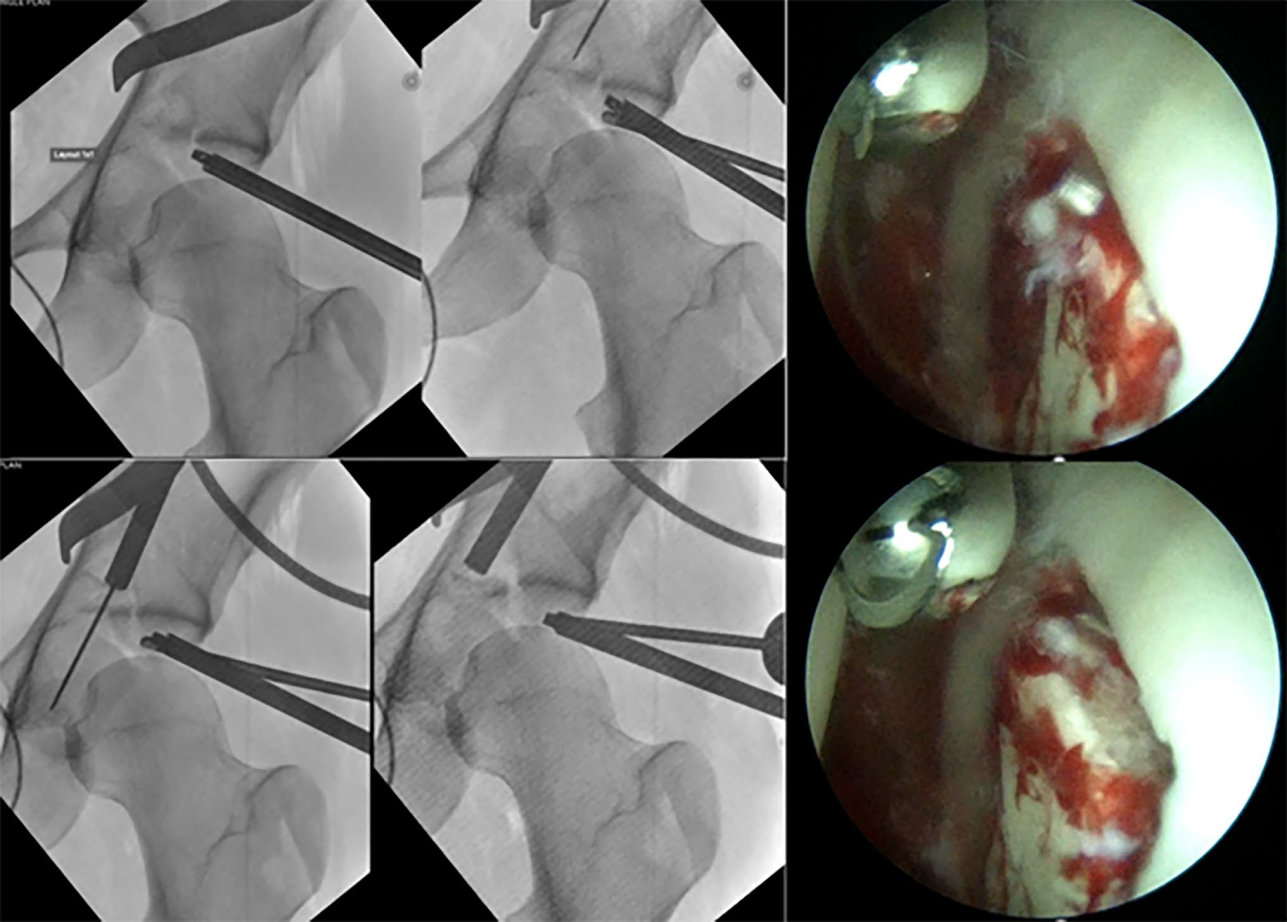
A healthy 21-year-old man presented to our ER after a crush trauma at work under a 500 kilos weight. The X-Ray and CT scan show an anterior column fracture plus posterior hemitransverse compound fracture (ACPHT) with joint depression of the acetabular roof. An AIP approach and hip arthroscopic assistance were performed. The patient was set up in supine position under general anesthesia on a carbon orthopedic traction bed. The fracture of the anterior column was identified through the AIP approach. The medial roof fracture and an important depression of the articular surface were identified through arthroscopic assistance. Supracetabular K-wire was placed under fluoroscopic control and proximal cortical flap was prepared to aid the fragment reduction. A cannulated ram was placed over the K wire and the depressed fragment was pushed stepwise until an anatomic articular reduction is achieved. At the end hypercorrection of the fragment was obtained as planned and synthetic bone was placed to fill the depression. The movement is continuously supervised by the arthroscope. Furthermore, the reduction of the fracture is evaluated by fluoroscopy. Surgery was performed in two hours and 30 min
